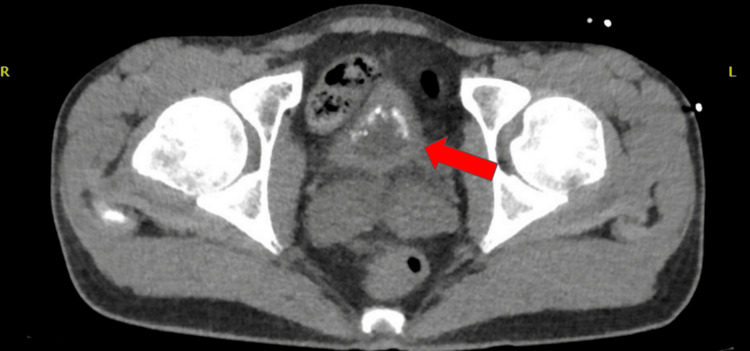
Axial CT (renal tract protocol) demonstrating bladder wall thickening with calcification (arrow).
